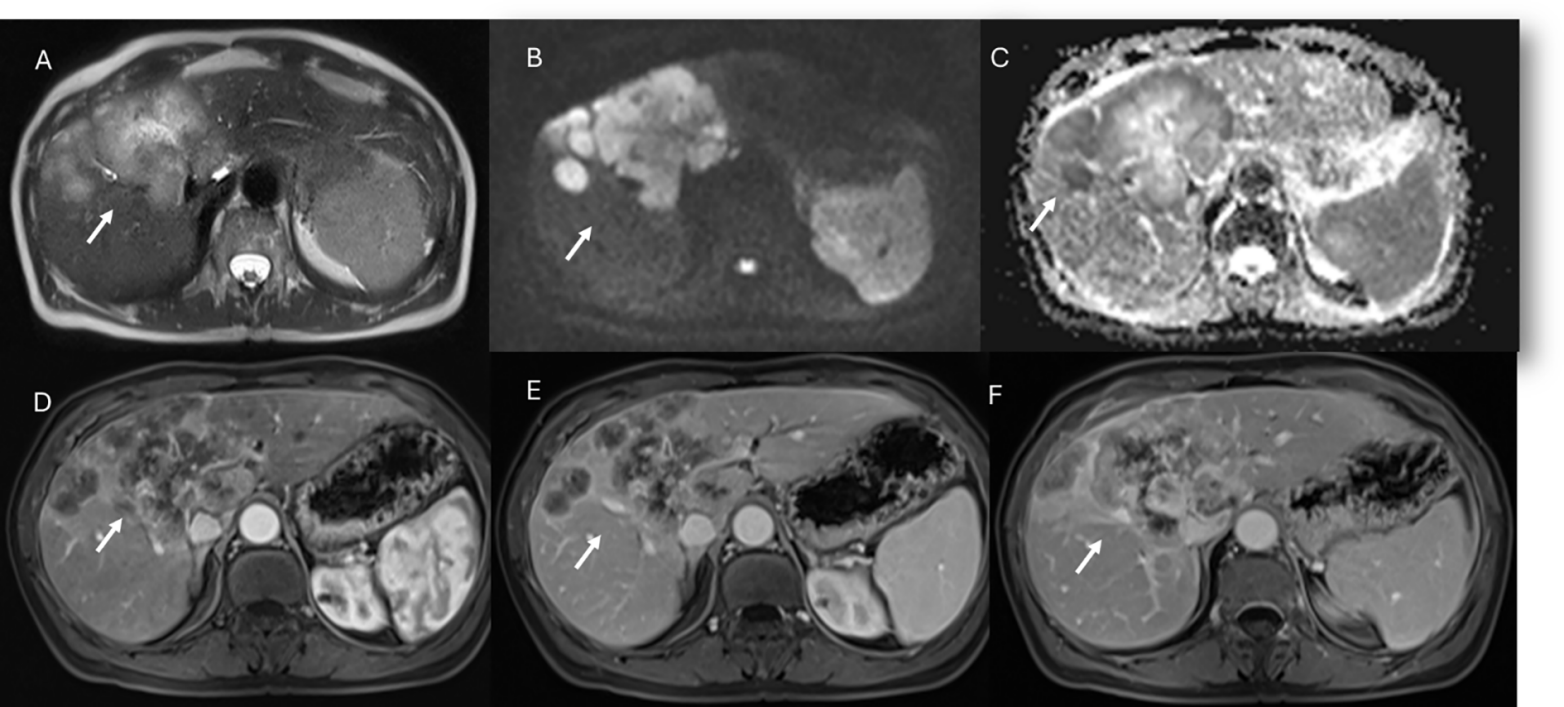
MRI assessment of non-mucinous colorectal liver metastases (arrows). In (A) (T2-W sequence, axial plane), the lesion (arrow) shows hyperintense signal with restricted diffusion on b = 880 s/mm² (B) and targetoid appearance on ADC map (C). After non-specific contrast agent, rim enhancement is seen in the arterial phase (D, T1-W, axial), with peripheral enhancement in the portal (E) and late phases (F) showing targetoid appearance from central necrosis.
